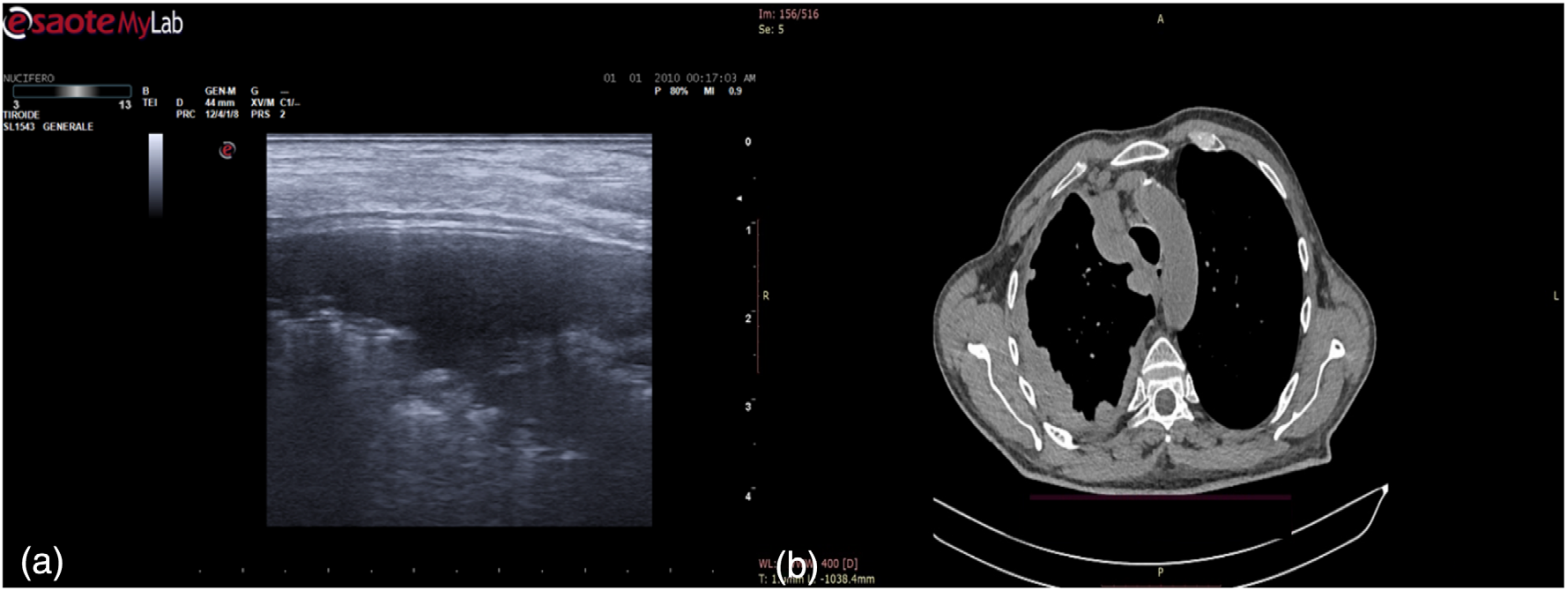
(a) Pleural thickening visualized as a lobular or oval hypoecogenic inhomogeneous structure, irregular shadow, hyperechoic spots and peripheral areas of these lesions may be characterized by an echo‐poor halo, central necrosis and spiculations. (b) Thoracic computed tomography (CT) shows circumferential pleural thickening.
